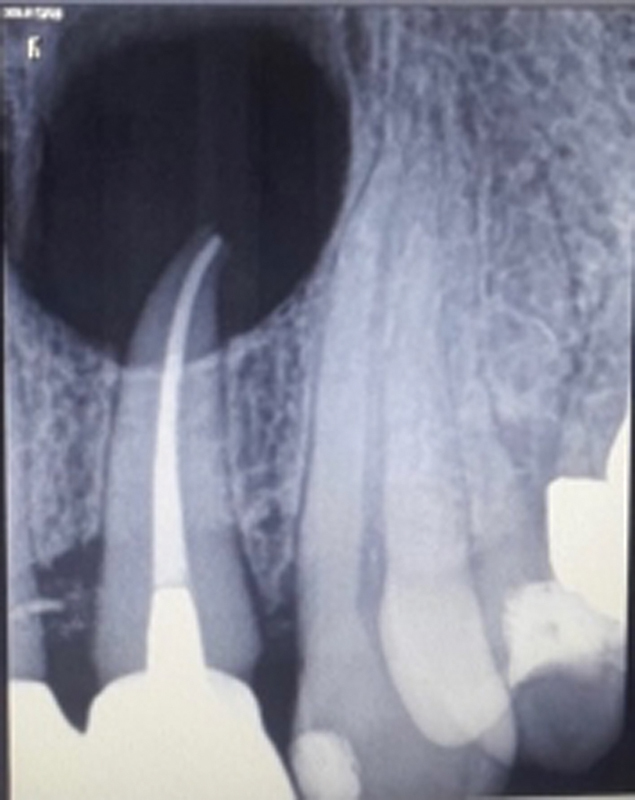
Periapical rx on #22 taken after root canal therapy.
